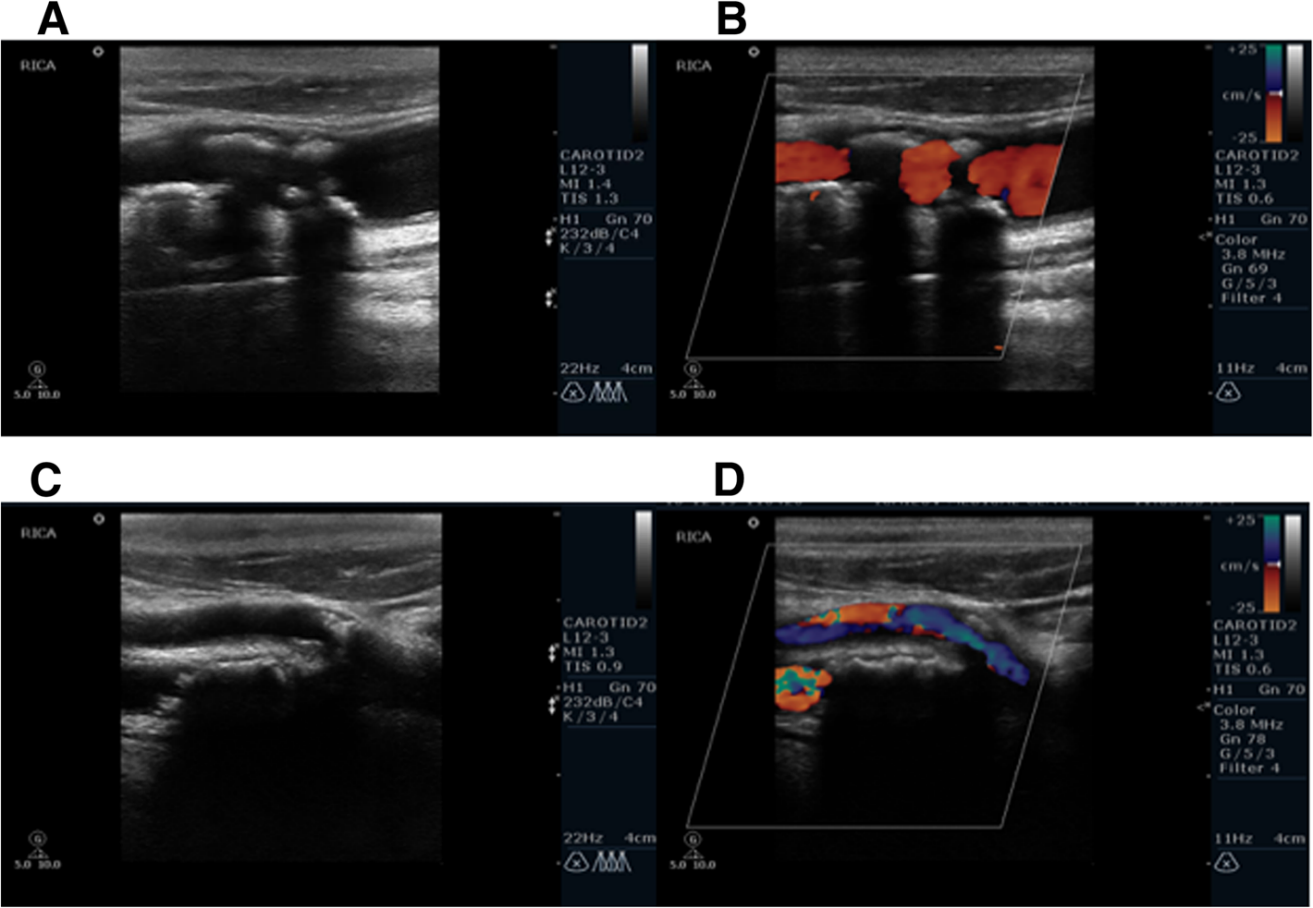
Acoustic Shadow on Sonographic imaging. Legend: Sonographic examples of a small (A + B) and large (C + D) AS (Acoustic shadow) artifact. B-mode ultrasonography of carotid bifurcation demonstrating an atherosclerotic plaque with a small (a) and large (c) AS artifact. Color Doppler ultrasonography images of carotid artery bifurcation demonstrating an atherosclerotic plaque with a small (b) and large (d) AS artifact
